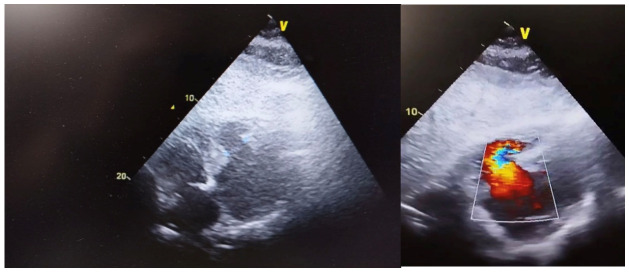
Echocardiography showing left-to-right shunt with color gradient.
